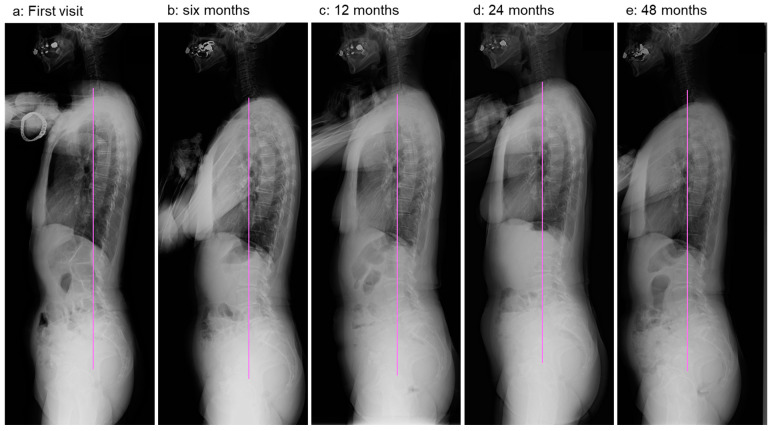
Standing lateral whole-spine radiographs at first visit (a), 6 months (b), 12 months (c), 24 months (d), and 48 months (e). Straight vertical line indicates C7PL.
